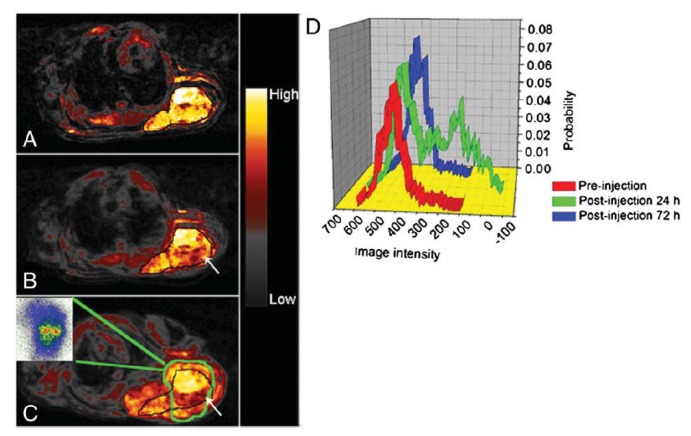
T2-weighted axial MRI for A431K5 tumors. (A), (B), and (C) show pre-injection and 24 and 72 h post-injection MR images. The inset represents autoradiographic images of 20 μM tumor sections; (D) ROI intensity histograms for T2-weighted axial gradient-echo MR images of A431K5 tumors, which show apparent shifts in a 24-h image towards low intensity. Reprinted with permission from [4].
